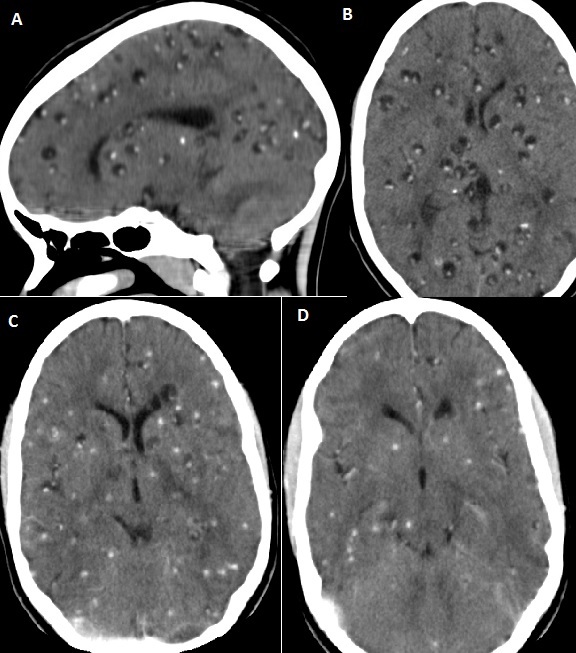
A) Brain CT(Sagittal view) showing Multiple Vesicular Cysts and Calcifications of Neurocysticercosis before treatment; B) Brain CT(axial view) showing Multiple Vesicular Cysts and Calcifications of Neurocysticercosis before treatment; C) Brain Ct (Axial View) showing resolution of almost all vesicular cysts with residual calcifications; D) Brain Ct after treatment
